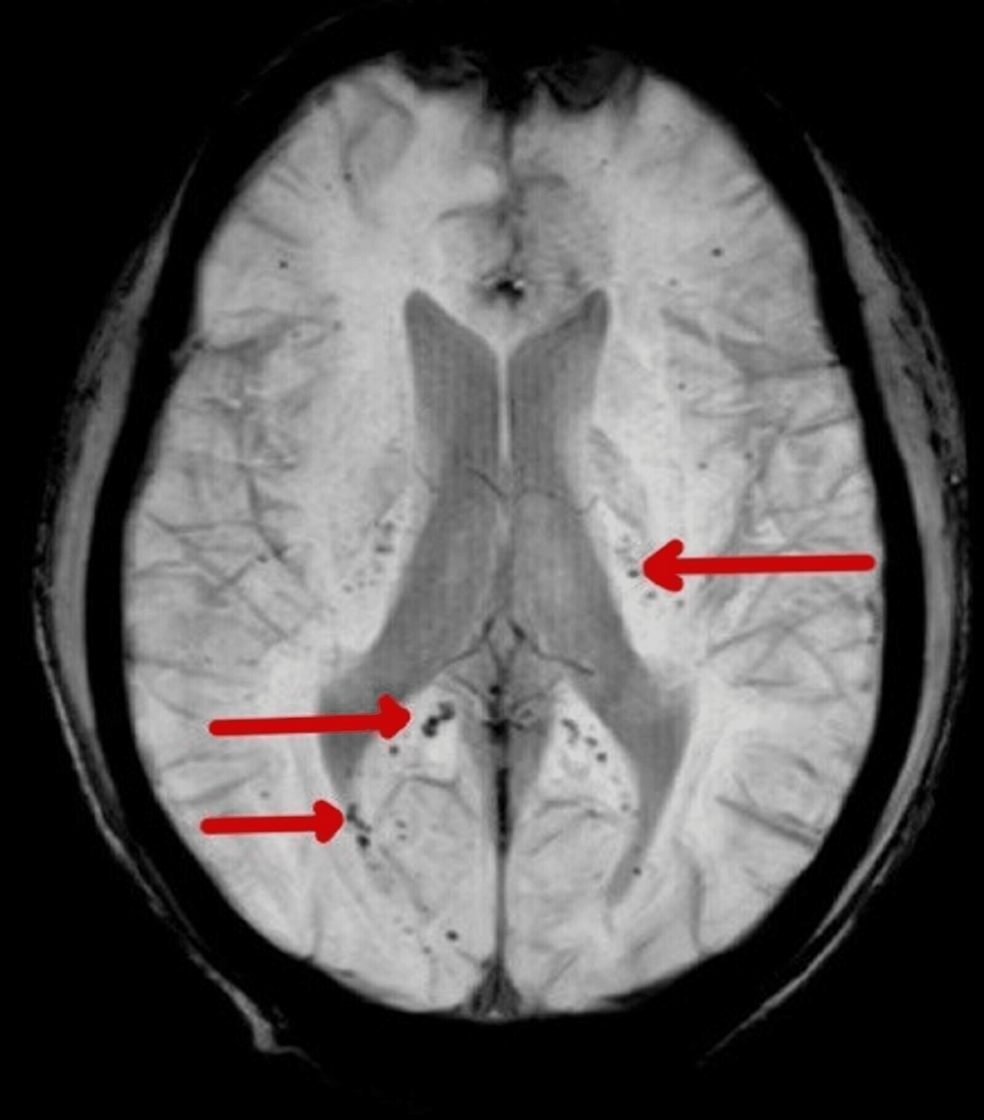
Susceptibility-weighted MRI shows multiple petechial hemorrhages involving bilateral halves of the splenium of the corpus callosum and bilateral internal capsule.
